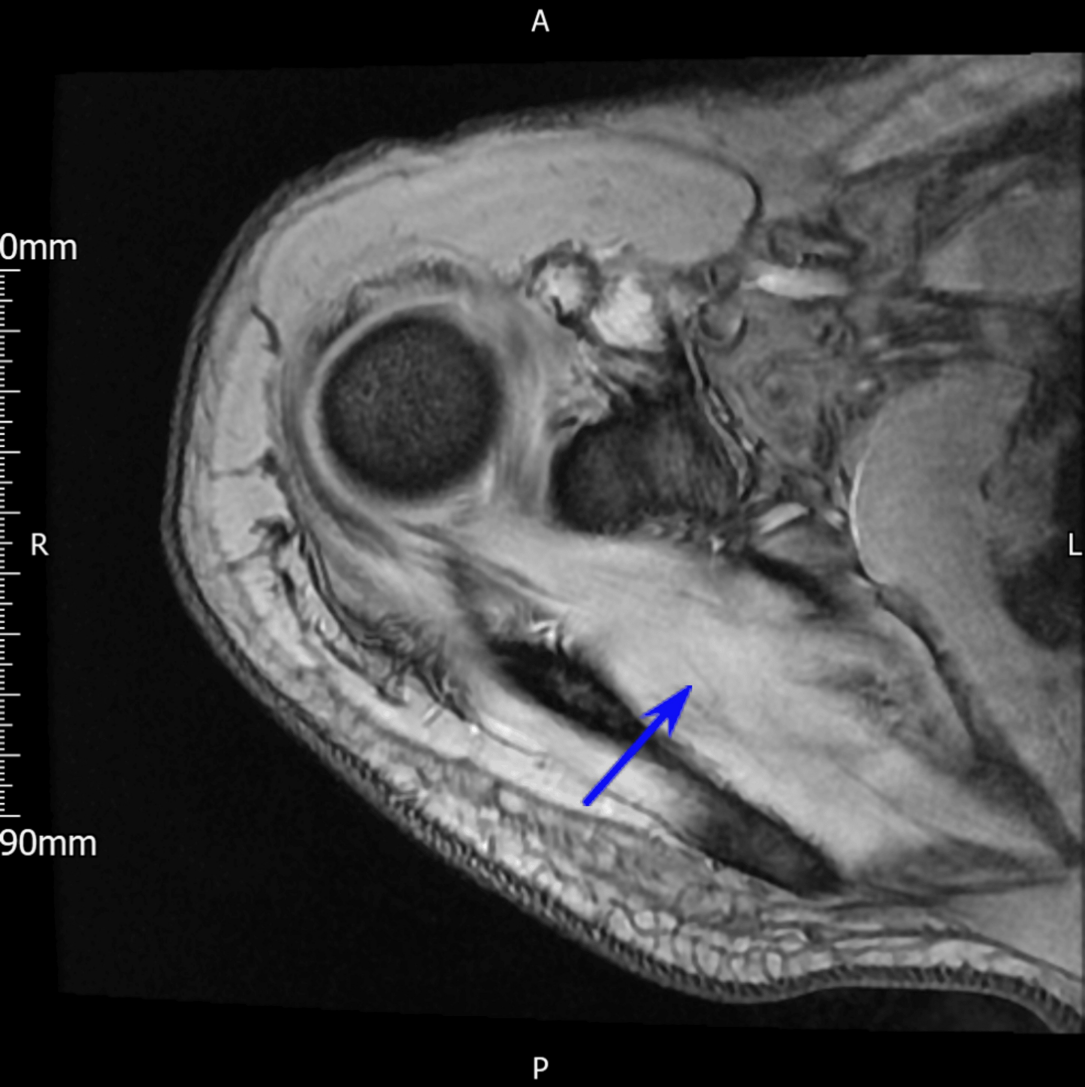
MRI axial 3D imaging of the coracoid mass. Arrow added to pinpoint the osseous mass.
